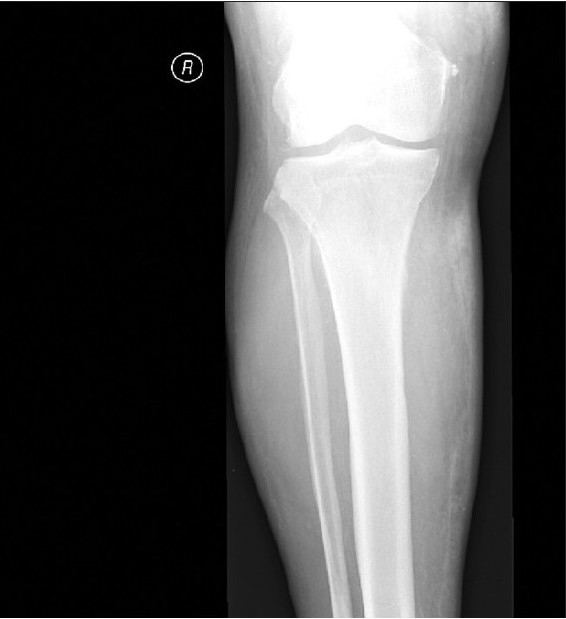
Soft tissue x-ray showed increased density on medial aspect of right lower leg
